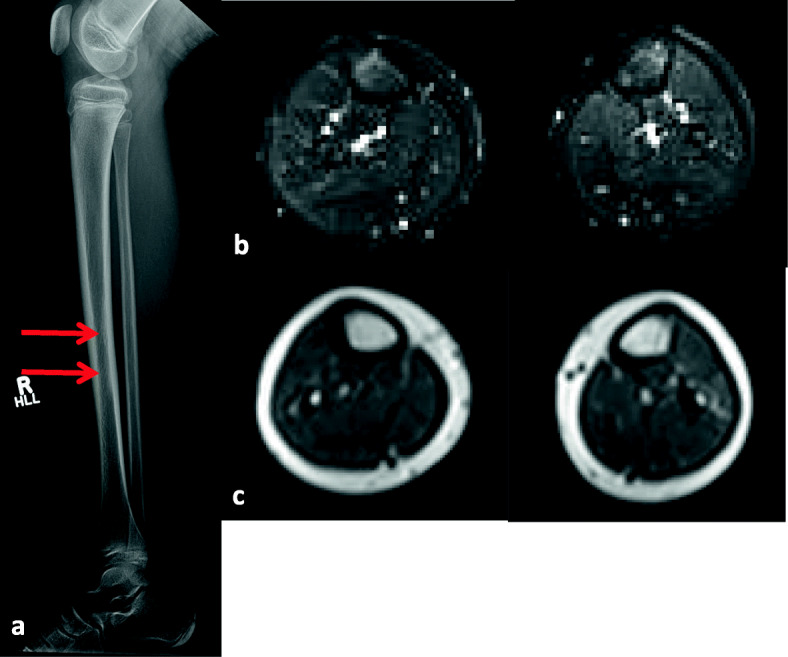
Case 6 (Follow-up) Follow-up lateral radiograph of the right lower extremity (a) obtained 3 months after the initial x-rays shows persistence of a focal area of sclerosis in the mid tibial diaphysis (arrows), increased in extent compared to previous. Subsequent axial inversion recovery (b) and T1 (c) MR images of the thighs obtained almost 1 year after the initial x-rays show interval resolution of the previously noted focus of increased bone marrow signal in the mid right tibial diaphysis
